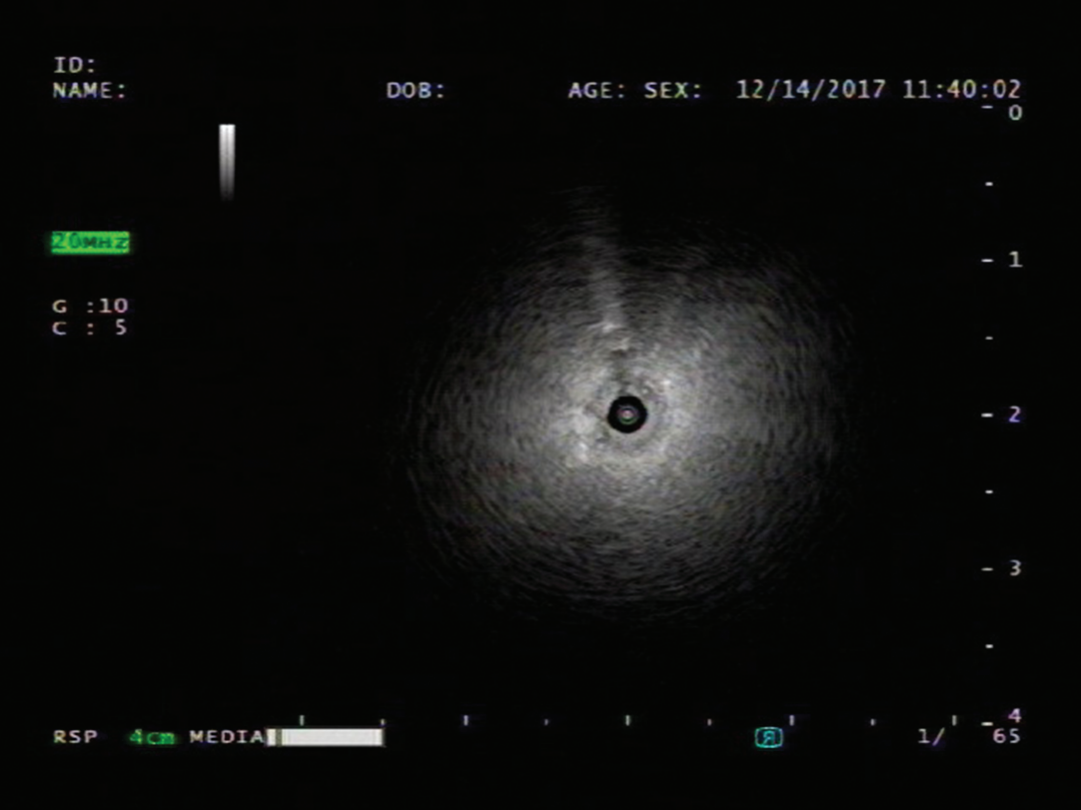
Radial probe endobronchial ultrasound (EBUS) showed a “blizzard sign”, suggesting ground‐glass opacity over the superior segment of right lower lobe.
